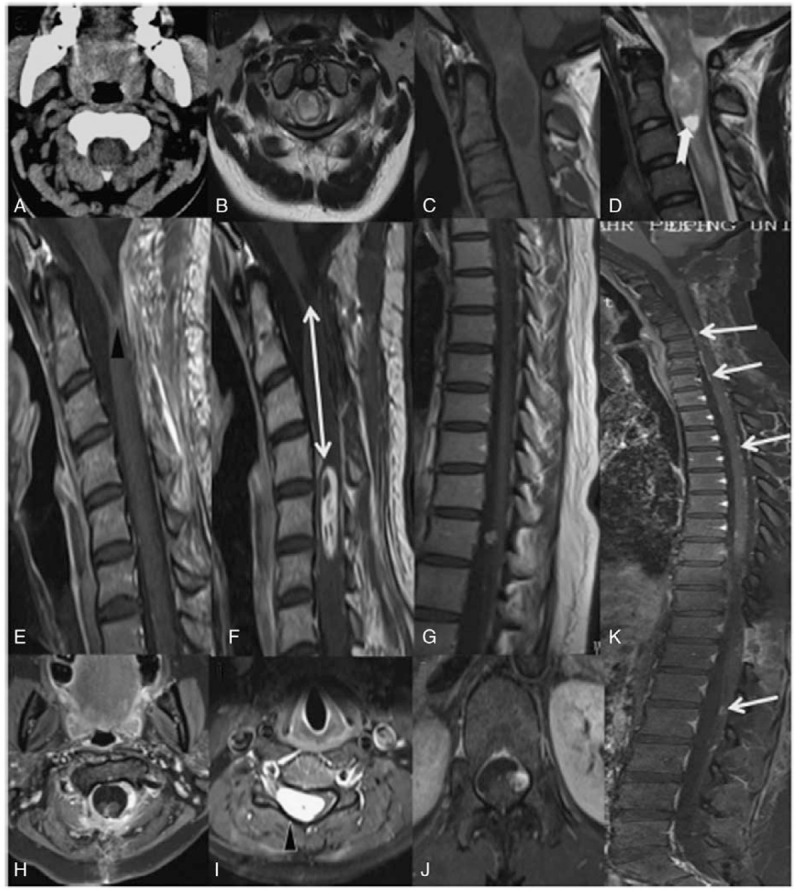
Preoperative plain computed tomography scan shows a hypodense lesion located at C1–C2 levels without calcification (A). Preoperative axial T1-weighted MRI shows the bad-delineated mass of hypointense signal located exclusively in the cervical spinal cord (B). Sagittal MRI (C and D) depicts the fusiform-shaped and eccentrically located intramedullary tumor at the C1–C2 levels, appearing hypointense on T1-weighted and mixed signal intensity on T2-weighted images with adjacent spinal cord edema. The cystic lesion within the spinal cord distal to the mass is quite visible (see white dovetail arrow). (E and H) Immediately postoperative Gd-enhanced T1-weighted sagittal and axial MRI shows gross-total removal of the tumor (black arrowhead). Sagittal (F and G) and axial (I and J) on the 10th month follow-up MRI images with contrast reveal two metastatic extramedullary lesions at C4–C6 and T11–12, respectively, appearing highly enhanced with heterogeneous features. No changes are observed in the previous operation site (see white double arrow). (K) Postoperative T1-weighted sagittal MRI with contrast depicts near-total resection of the cranial and caudal tumors, respectively. Of note, numerous additional disseminated enhancing foci nodules are seen along the leptomeninges and dura, likely representing metastatic drop lesions (long white arrows). (Han Z, Du Y, Qi H, Zheng S, Yin W. Cervical intramedullary immature teratoma with metastatic recurrence in an adult. Spinal Cord Ser Cases. 2015;1:15006.).
